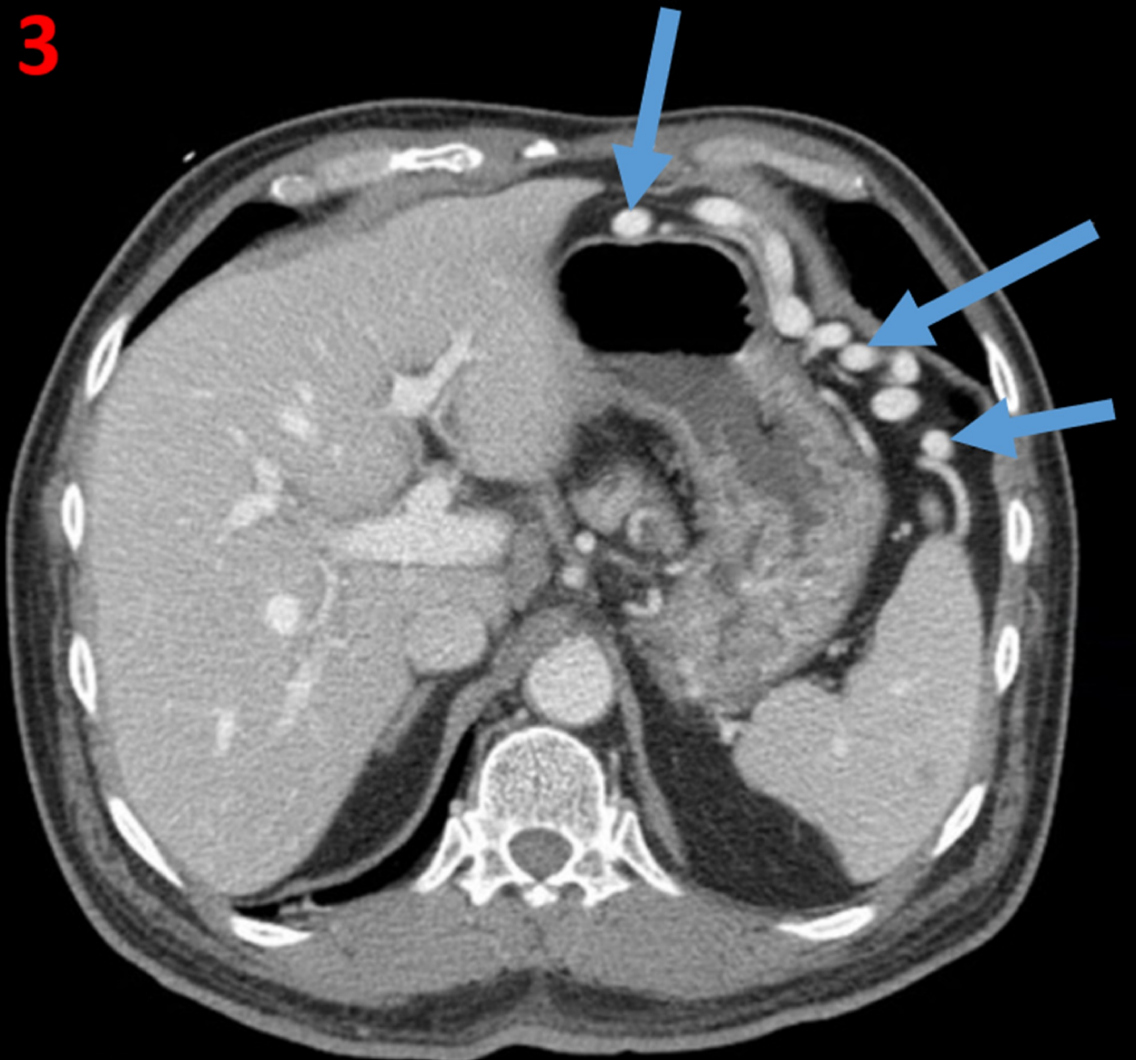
Varix on axial abdominal CT CT: Computed tomography Axial CT demonstrates a large collateral varix (blue arrows) which courses through abdominal mesentery. This varix is providing venous drainage of the spleen due to chronic splenic vein thrombosis
